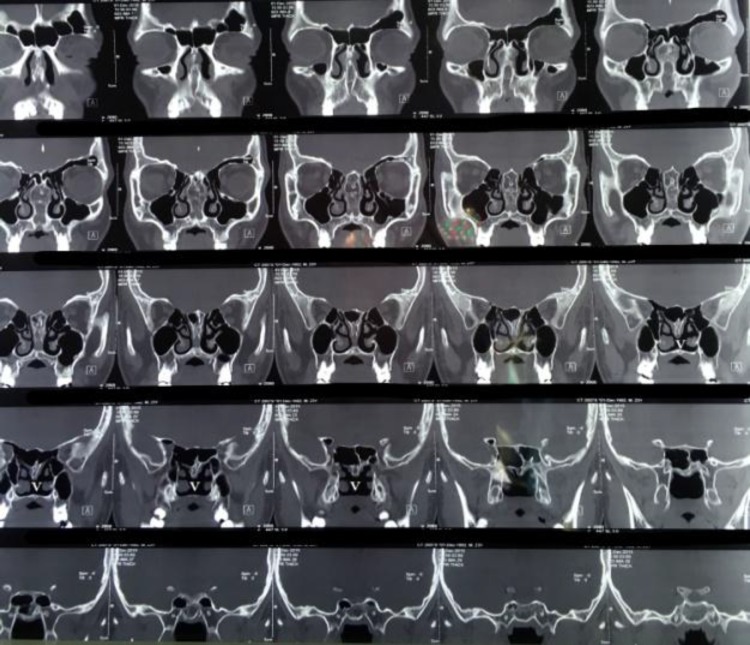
CT scan of case 2 that shows posteroinferior nasal septum defect in proposed vomerian part (V) and also thickening of the rest of septum
